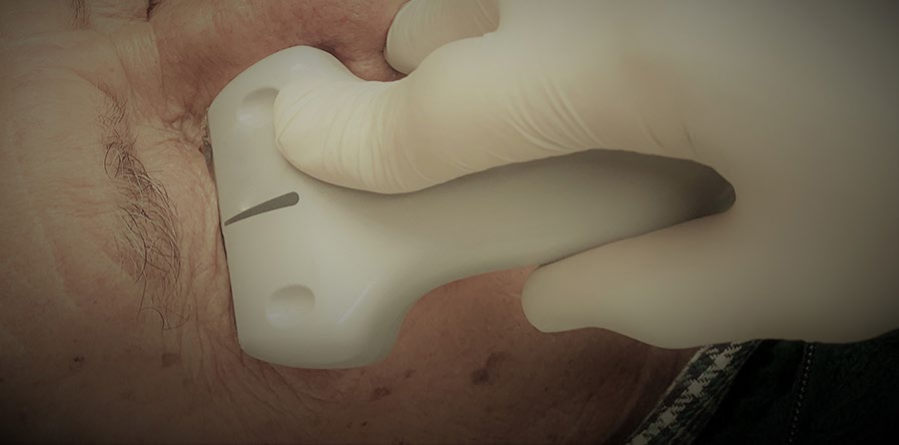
Ultrasonographic pupillary assessment. The 7.5–15 MHz linear transducer gently placed over the lower edge of the closed eye for a trans-palpebral tangential view
